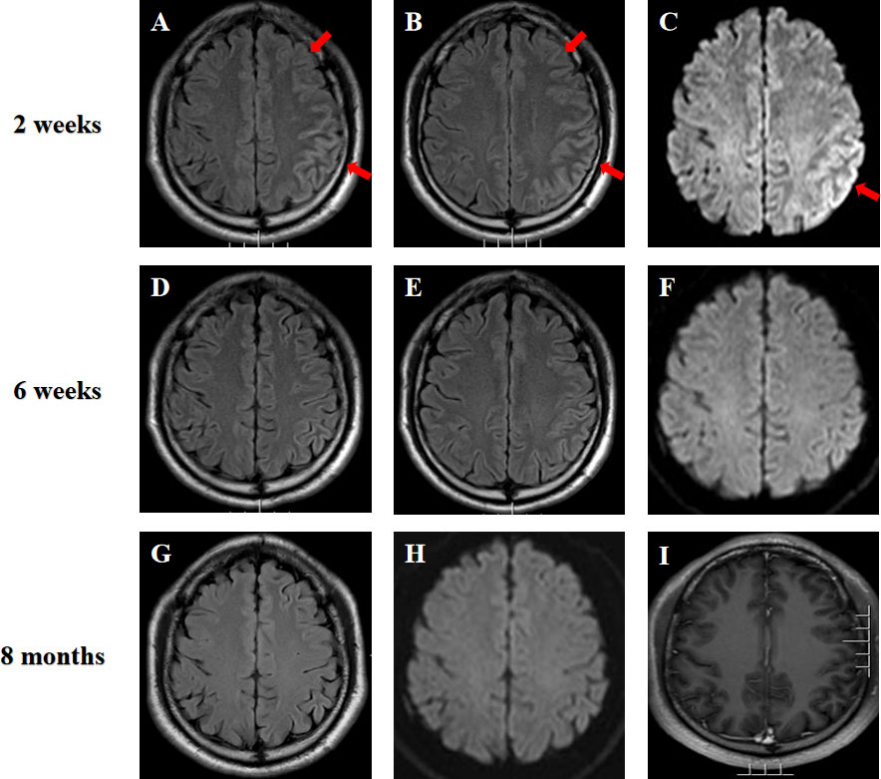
(A–C) Brain MRI of the patient at two weeks after the symptom onset showed hyperintensity of the extensive cortex in left cerebral hemisphere on fluid attenuated inversion recovery (FLAIR) imaging and diffusion weighted imaging (DWI) (arrows). (D–F) MRI was repeated at six weeks and showed a significant improvement of the imaging abnormality. (G–I) MRI performed at almost eight months after initial symptom onset depicted no signal abnormalities on FLAIR, DWI and T1-weighted gadolinium-enhanced sequences.
